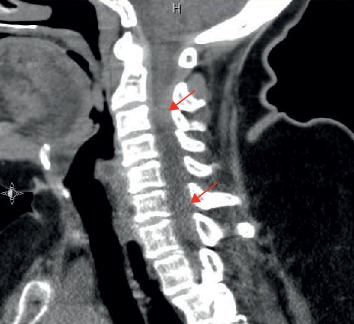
Sagittal CT scan image showing posterior epidural hematoma.
